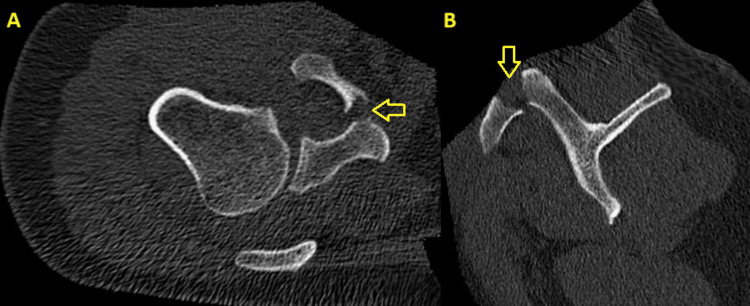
Preoperative CT scan. The yellow arrows highlight the displaced coracoid process fracture. (A) Transverse view. (B) Sagittal view.
